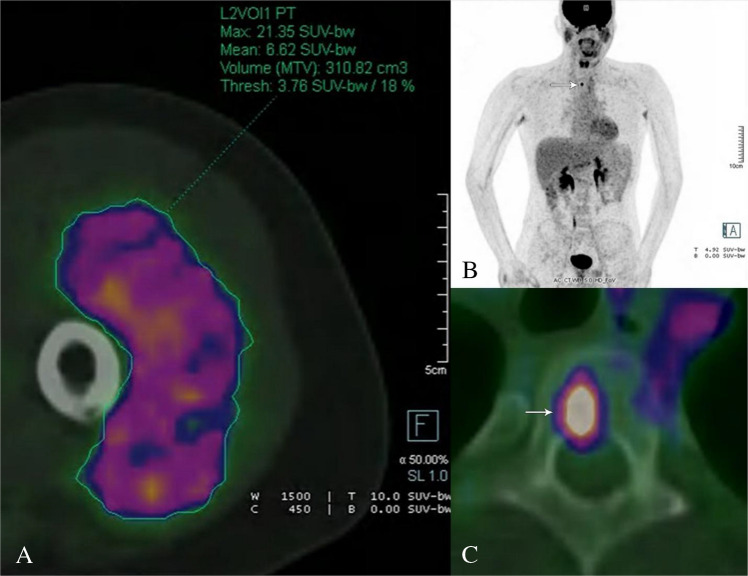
20-year-old male with Ewing sarcoma of the left femur diaphysis with a large extraskeletal component. Baseline axial ^18^F-FDG PET-CT (A) showed large dimensions (max diameter 11 cm, vol. > 200 ml), high SUV (max 21, mean 6.6). The patient presented with a single skeletal metastasis at diagnosis of the T3 thoracic vertebral body on whole-body MIP (B) and axial PET-CT (C) (arrows)
